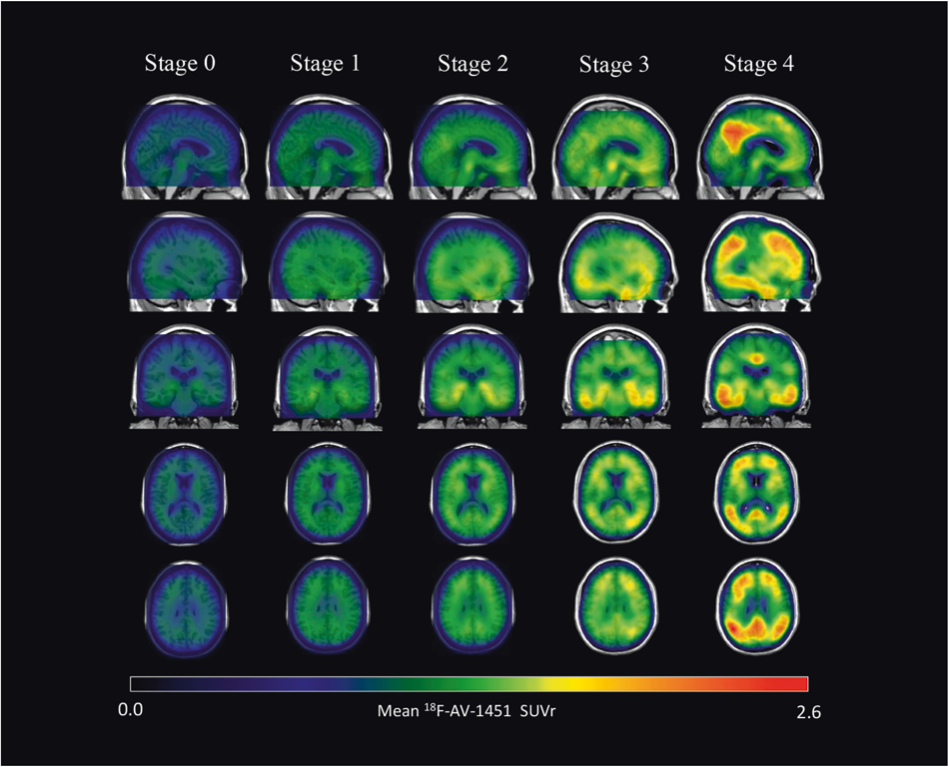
Parametric ^18^F-AV-1451 images across stages. In general, ^18^F-AV-1451 SUVr increased throughout the cortex and subcortex from stage 0 to stage 4 (numerical values shown in Table 1). Participants in stage 0 had tau levels corresponding to those of normal young adults. A dominating tau elevation in medial temporal regions (Braak I/II ROIs) was shown in stage 1. While stage 2 presented increased SUVrs in extra-medial temporal regions, stage 3 showed greater SUVrs increase in Braak III/IV ROIs including inferior and lateral temporal lobes. Stage 4 had significantly elevated ^18^F-AV-1451 SUVr extending into the neocortex. ROI region of interest, SUVr standard uptake value ratio.
